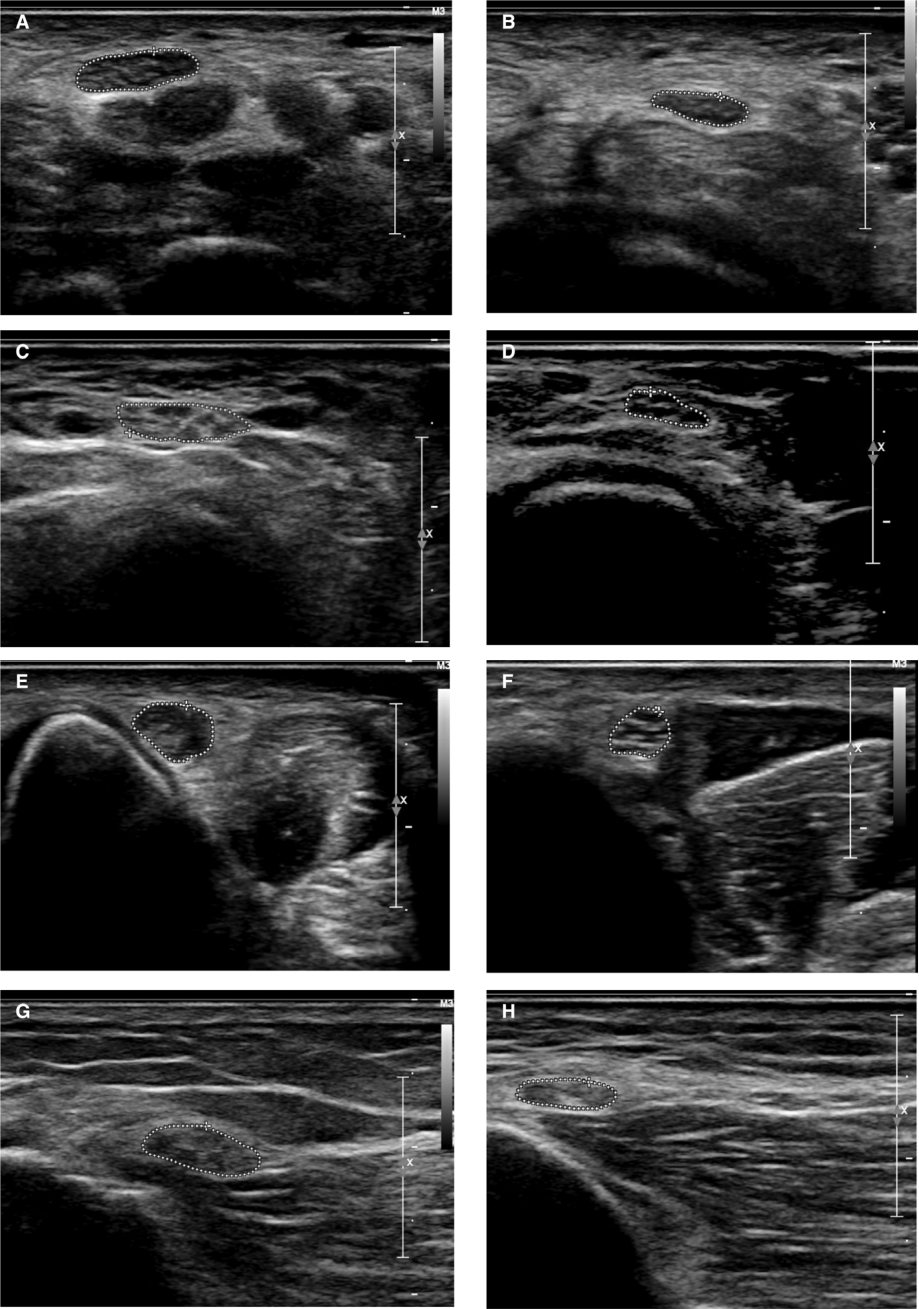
(A–H) High‐resolution ultrasound of peripheral nerves at entrapment sites: PD patients (A, C, E, and G; for all *n* = 45) versus controls (B, D, F, and H; for all *n* = 75): A and B: median nerve: carpal tunnel. (C and D) Ulnar nerve: Loge de Guyon. (E and F) Ulnar nerve: sulcus. (G and H) Fibular nerve: head. PD, Parkinson’s disease.
